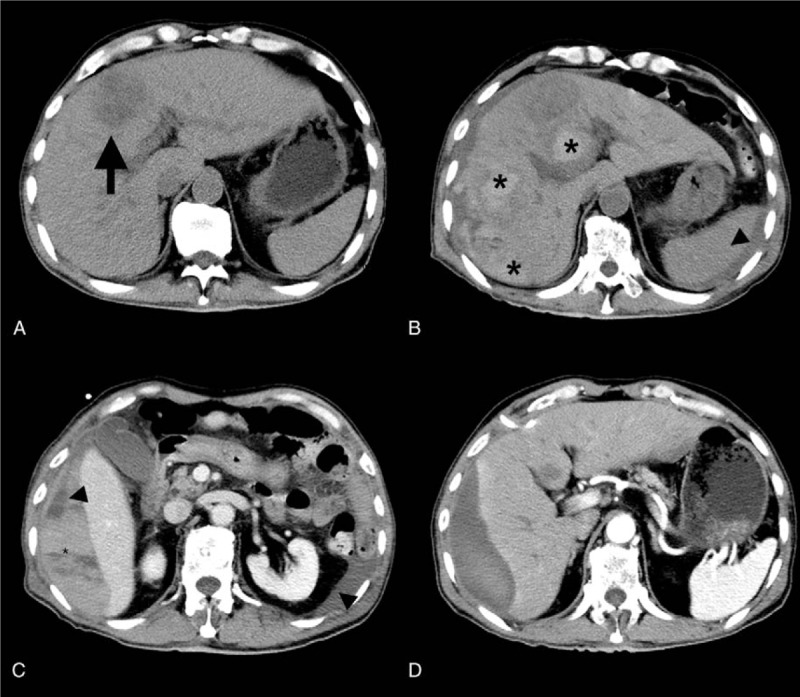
CT images before and after PCD. A. CT images on 3 days before PCD: a 4 cm liver abscess located in segment IV (arrow). B, C. CT images on 2 days after PCD: subcapsular and intrahepatic hematoma (asterisk), subcapsular and intraabdominal hypo-intense fluid collection (arrowhead), residual liver abscess and drainage catheter shedding. D. CT images on 34 days after PCD: repeated CT revealed hematoma and ascites had been absorbed significantly, abscess cavity closure with residual subcapsular fluid accumulation. CT = computed tomography, PCD = percutaneous catheter drainage.
